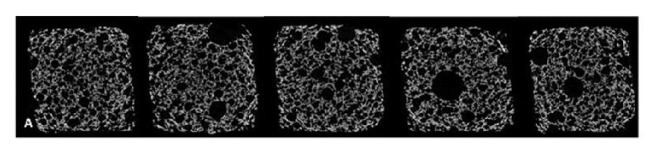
X-ray microtomography 2D reconstructed cross section images of cake samples [11].
